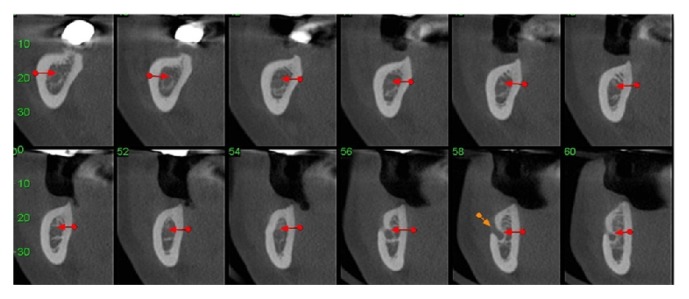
Computed tomography (cone beam) of the jaw, right side.
